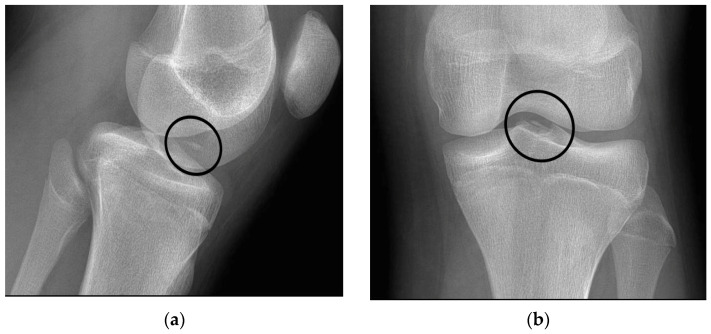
X-ray radiographs of the patient in two planes, (a) anteroposterior (AP) view and (b) lateral (L) view, of the injured knee. The fractured fragment is highlighted in the figure.
